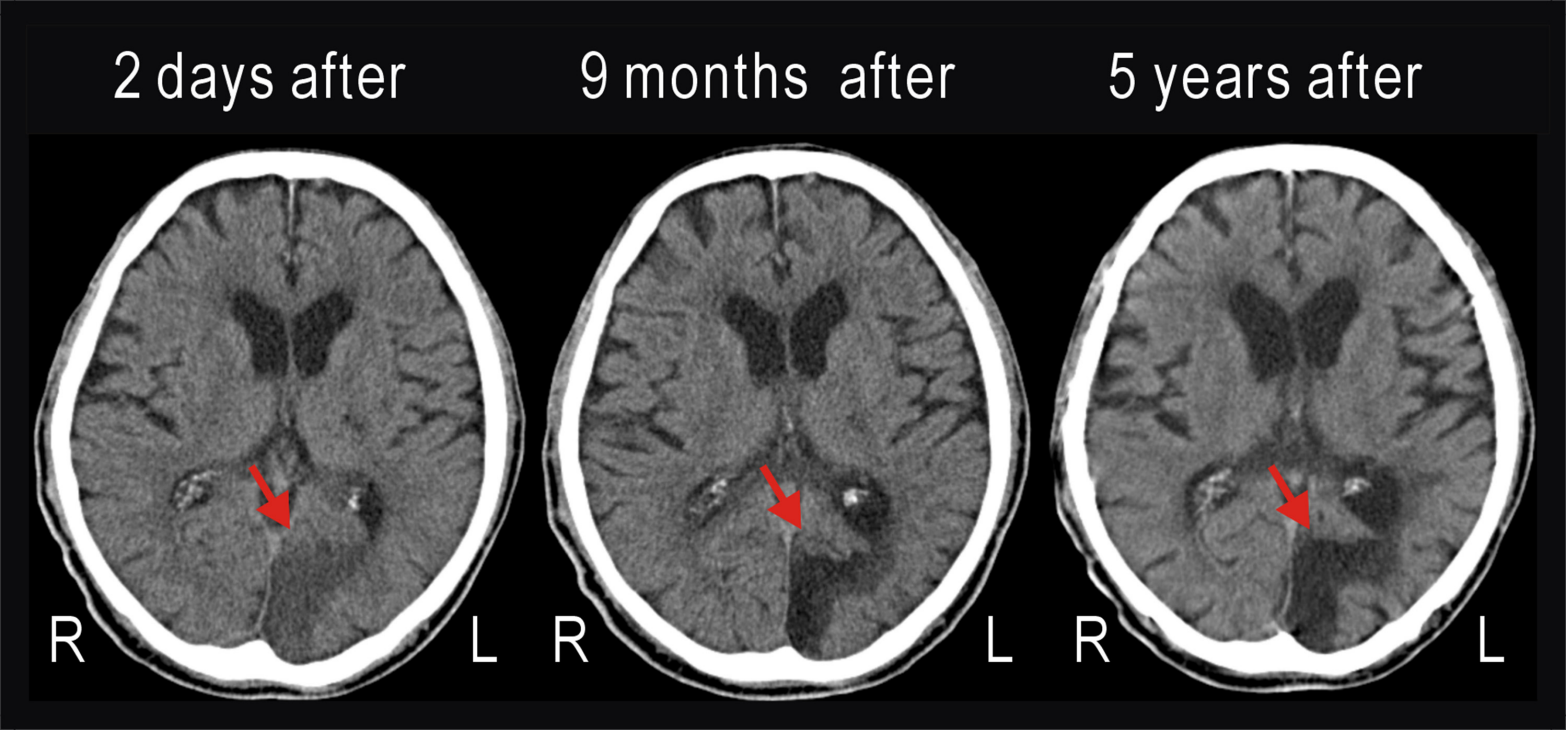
Computed tomography (CT) images taken 2 days, 9 months, and 5 years after cerebral infarction had occurred in the left occipital lobe of the patient. The infarction (red arrow) was unchanged during 5 years.
